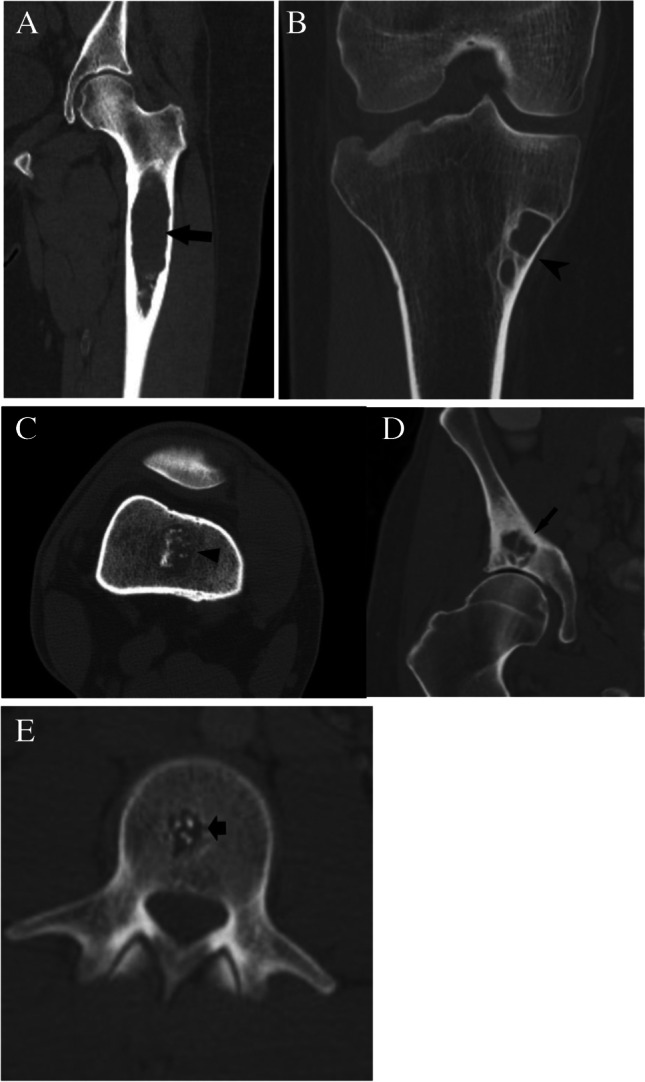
Characteristic CT lucent lesions. A Coronal CT image shows a lucent lesion (arrow) in the proximal femur consistent with fibrous dysplasia, B coronal CT image shows a cortically based lucent non-ossifying fibroma in the proximal tibia, C coronal CT image shows an enchondroma with punctate calcifications in the distal femur, D coronal CT image shows a subchondral cyst abutting the right hip joint with degenerative changes, and E axial CT image shows a lucent lesion with a “polka dot” appearance of calcifications in T12 consistent with a hemangioma
